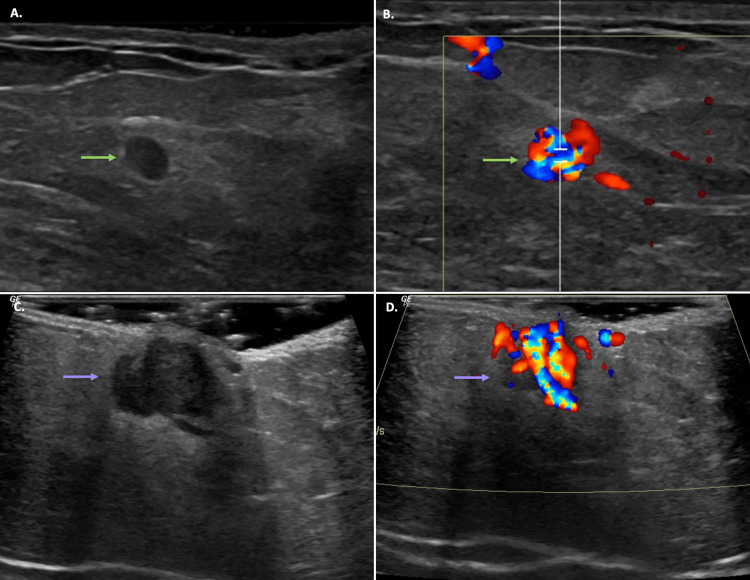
Left Breast and Axillary Ultrasound Findings (A, B) Hypoechoic, circumscribed, round, hypervascular mass (lime green arrows) is seen in the left breast at 10:00, 3-4 cm from the nipple, which corresponded to the mass seen on mammogram. (C, D) A second, larger, more superficial mass (lavender arrows) is seen in the left axilla. The left axillary mass was hypoechoic and hypervascular, similar in appearance to the 10 o' clock left breast mass.
